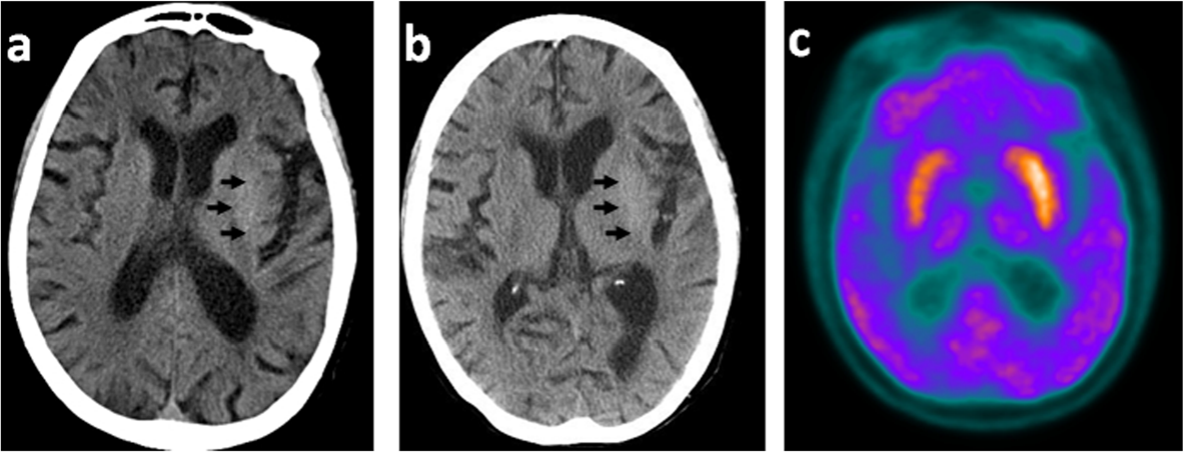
Images from patient 1. a. Brain CT (axial) the same day of onset, showing a hyperdense lesion in the left putamen (arrow); b. Brain CT(axial) 4 days after onset, revealing a slight high-density in the same area, but the density decreased compared with the initial CT (arrow); c. The FDG-PET scan (axial) 9 days after onset, revealing hyper-perfusion in the left side of the basal ganglion.
